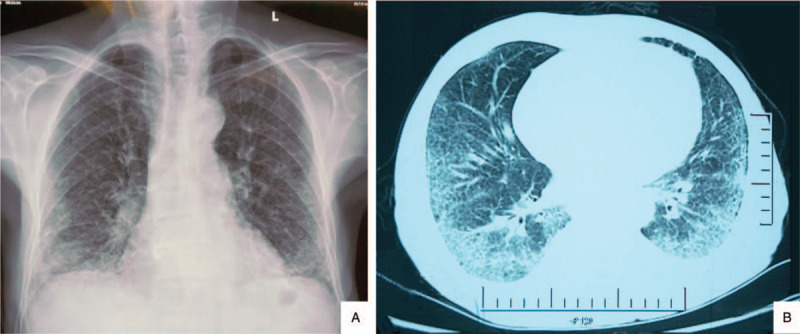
Imaging features. Chest X-ray(A) and CT(B) showed pulmonary interstitial fibrosis.
